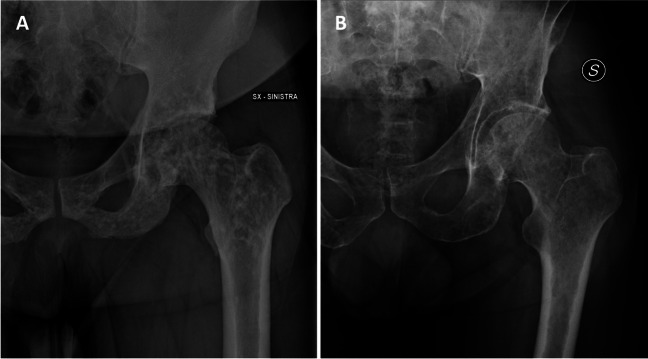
Radiographic examples of multiple sclerotic lesions (a osteopoikilosis) and multiple lytic lesions (b multiple myeloma) of the pelvis
